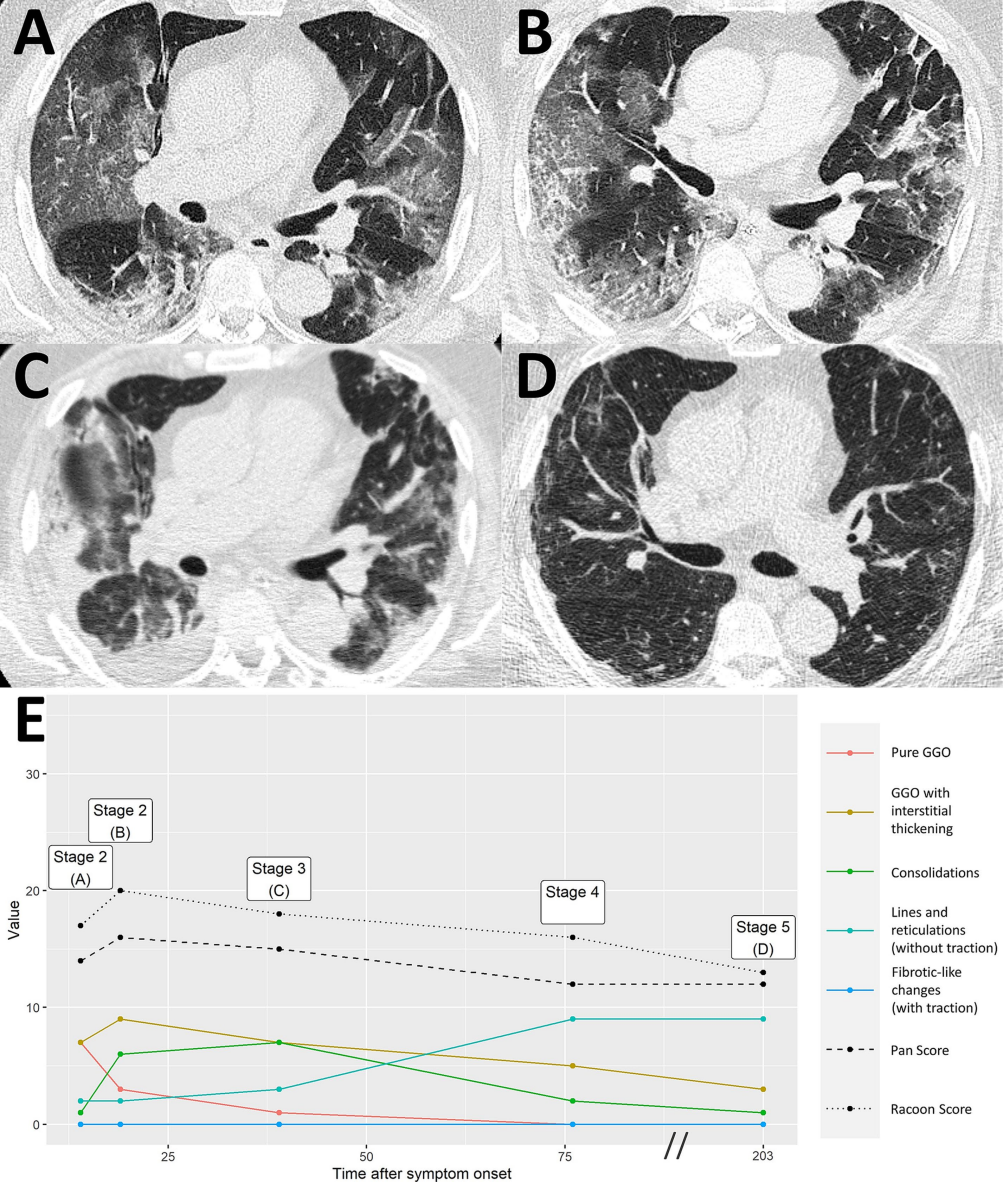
Severe COVID-19 pneumonia with residual imaging findings after >6 months follow-up. A 64-year-old male patient presented at the hospital 14 days after symptom onset, suffering from headaches and difficulty breathing. Chest CT demonstrated extensive ground glass opacities (GGO) with beginning interstitial thickening, consistent with progressive stage COVID-19 pneumonia [A, RVPS = 17 (7/7/1/2/0); stage 2]. Another chest CT 19 days after symptom onset reported an advance of the pneumonia to predominantly interstitial pathology and less ground glass [B, RVPS = 20 (3/9/6/2/0); stage 2]. Peak stage COVID-19 pneumonia with maximum consolidation was reached at 39 days after symptom onset [C, RVPS = 18 (1/7/7/3/0); stage 3]. Follow-up imaging on day 203 after symptom onset showed increasing linear opacities and fine parenchymal bands, yet no fibrotic-like changes with traction [D, RVPS = 13 (0/3/1/9/0); stage 5]. The subscores of the RVPS enable appreciation of such a typical time course of COVID-19 pneumonia, which is demonstrated in (E): Early and progressive stages are dominated by GGO and interstitial pathology (red/yellow), followed by a maximum of consolidation in peak stage (green), and eventually formation of linear opacities in resorption and post-COVID-19 stages (turquoise). RVPS, RACOON Viral Pneumonia Score; GGO, ground glass opacities.
